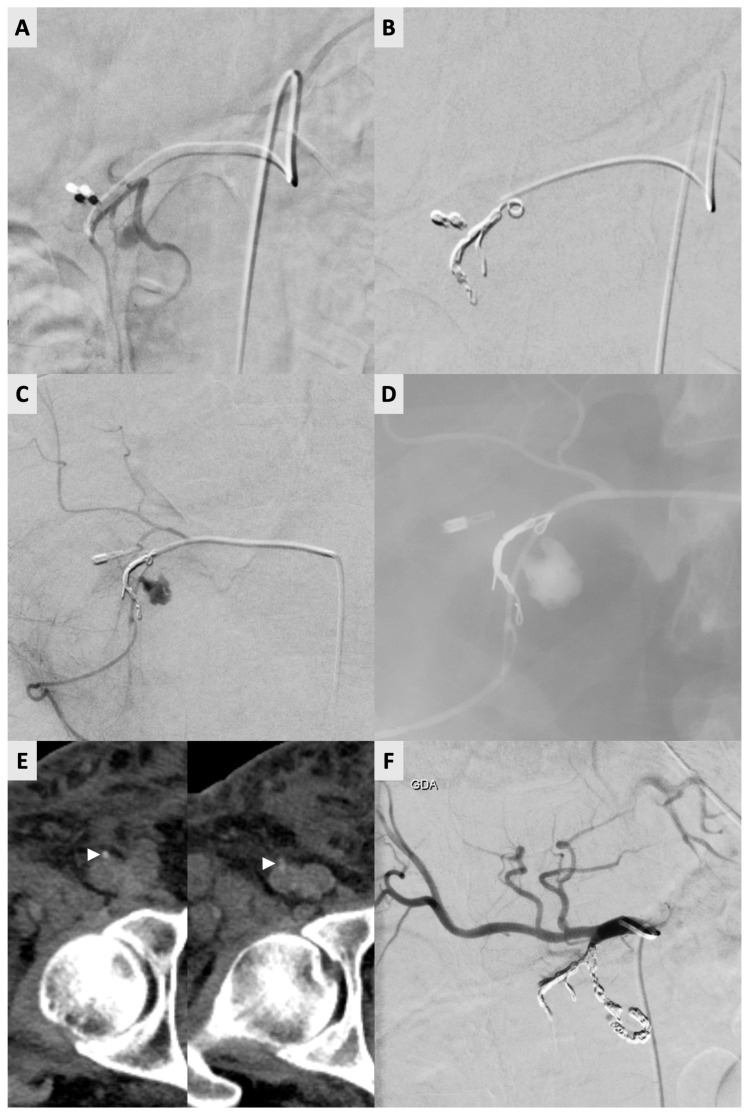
A 60-year-old male undergoing endoscopic ultrasound-guided fine needle aspiration (EUS-FNA) for a pancreatic mass, complicated by duodenal hemorrhage, requiring angiographic intervention. (A) Initial angiogram revealing a pseudoaneurysm of the posterior superior pancreaticoduodenal artery (PSPDA). (B) Successful coil embolization of the gastroduodenal artery (GDA) and PSPDA was performed with favorable angiographic outcome. Hemostasis was achieved with 6F AngioSeal. (C) Recurrent symptoms 4 days after index procedure prompted a repeat angiogram confirming recanalization of the pseudoaneurysm. (D) Subsequent embolization of the pseudoaneurysm using glue was performed. Hemostasis again achieved with 6F AngioSeal. (E) Symptoms recurred 3 days later, and a follow-up CTconfirmed re-bleeding. Unenhanced CT of the right common femoral artery (CFA) showing two closely positioned AngioSeal devices (white arrowheads) seen as small high-density structures within or adjacent to the previous puncture. (F) Re-repeat definitive embolization of GDA branches with coils to address persistent bleeding was performed and patient’s bleeding symptoms resolved. Hemostasis was again achieved with 6F AngioSeal, the 3rd deployment in the same vessel in 8 days.
